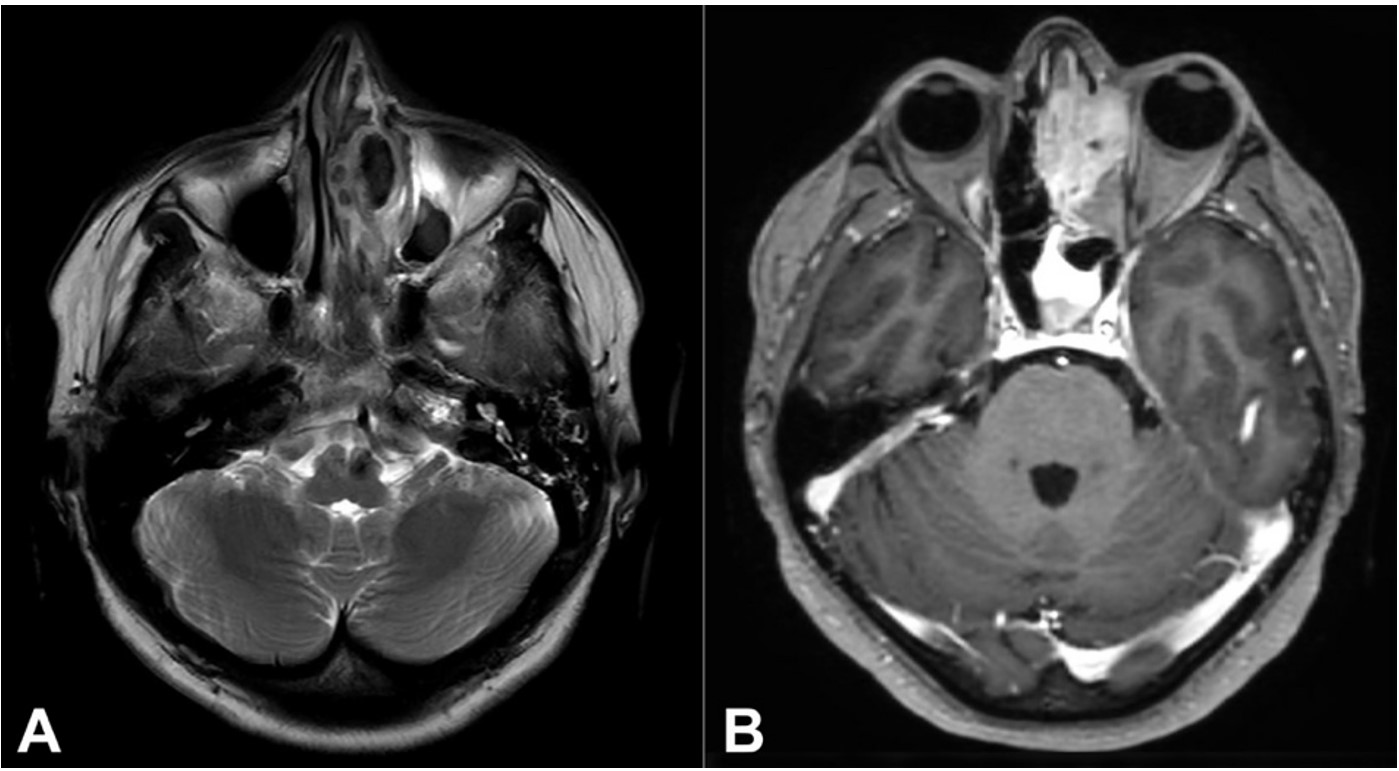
MRI imaging showed: A - heterogeneous mass on T2W1; B - hemosinus was observed in the left sphenoid sinus.
